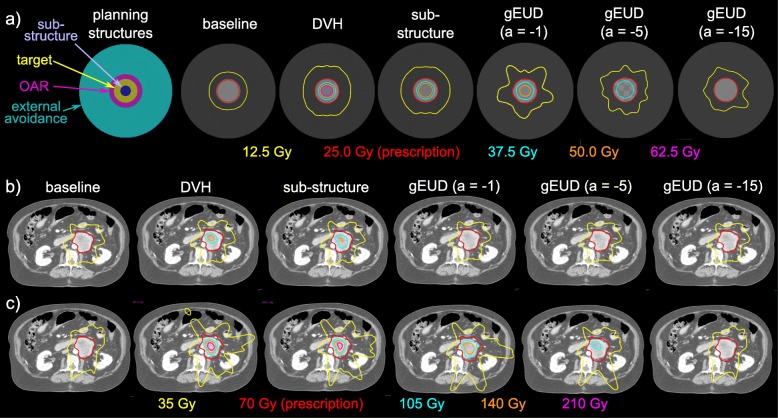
a) Axial views of the virtual CT, planning structures, and isodose lines for each planning approach for the 5 cm tumor diameter. b) Axial views of the patient plans optimized using each planning approach while meeting all OAR constraints and an R50% < 3.4. c) Axial views of the patient plans optimized using each approach while meeting all OAR constraints and relaxing the dose objectives limiting the R50%
